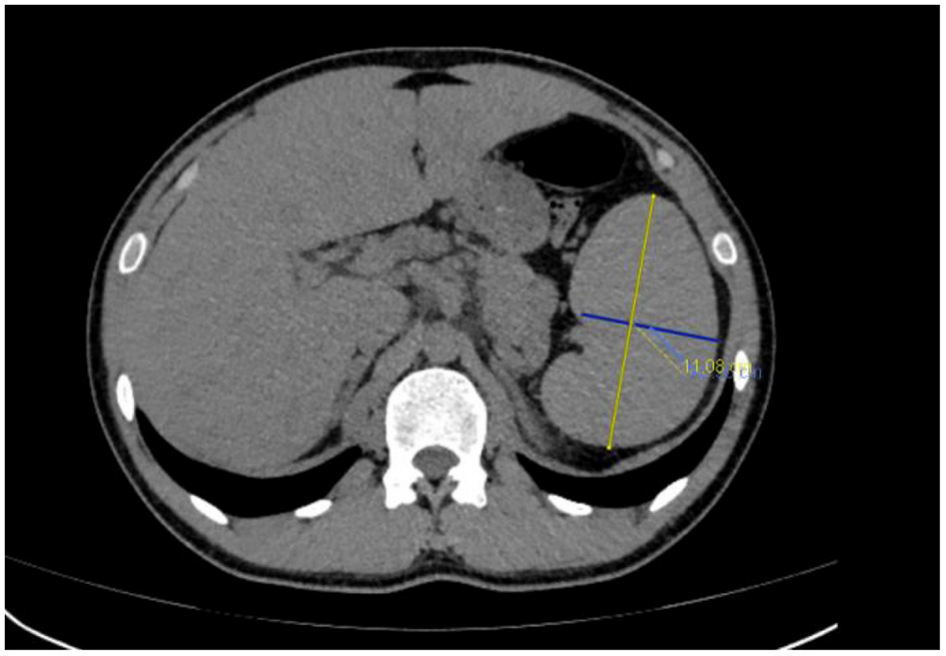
Abdominal and computed tomography (CT) revealed splenomegaly.
